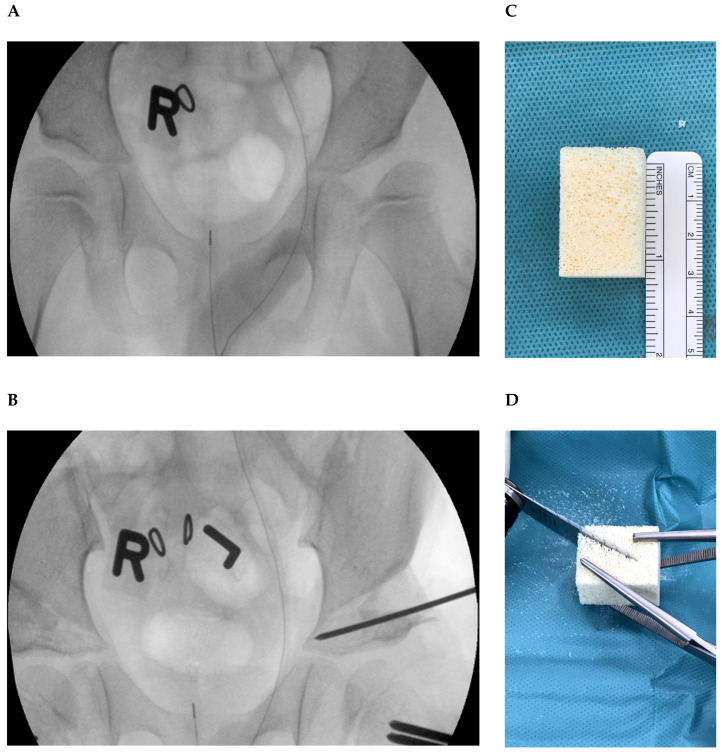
Intraoperative anteroposterior radiograph of the pelvis of a 7-years old boy with bilateral acetabular dysplasia and coxa valga before (A) and after (B) Dega osteotomy and proximal femoral varus derotation osteotomy of both hips. A Tutobone^®^ block (C) was cut into a triangular shape (D) and inserted to stabilize the new acetabular position.
